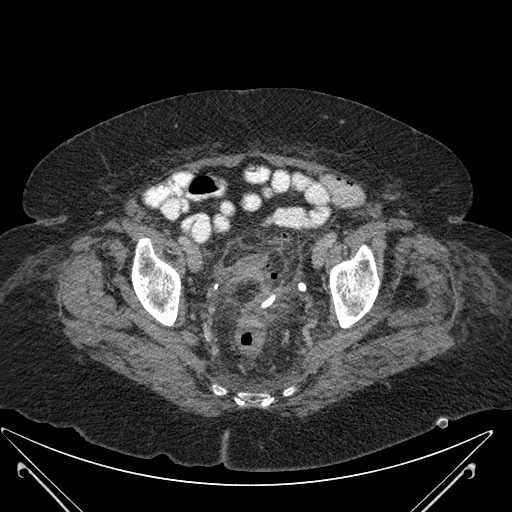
Computed tomography scan of the abdomen and pelvis (axial section) after drainage of the abscess with catheter *in-situ*.
